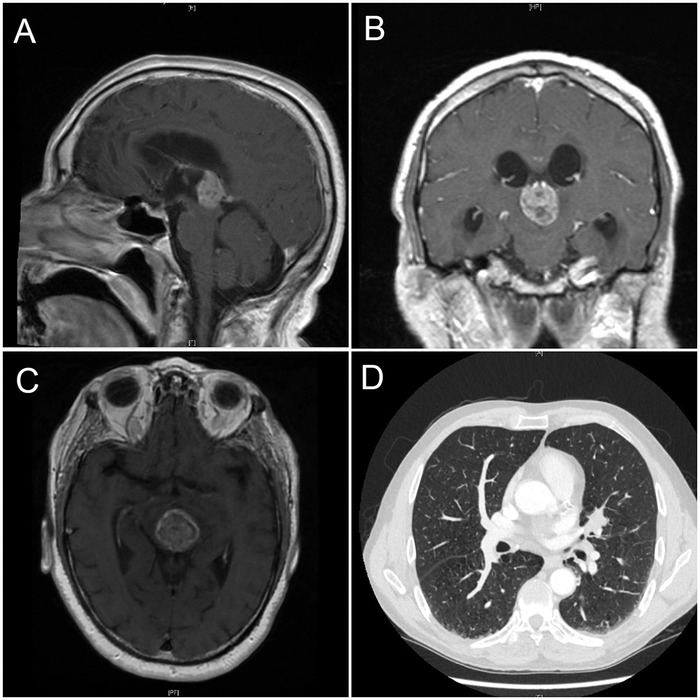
(A) Sagittal sections of magnetic resonance imaging (MRI) of the brain showing the pineal gland mass. (B) Coronal and (C) transverse sections of brain MRI showing the same pineal tumor. (D) Computed tomography of the chest with intravenous contrast showing a lung nodule over the left hilar region in the lower right corner.
